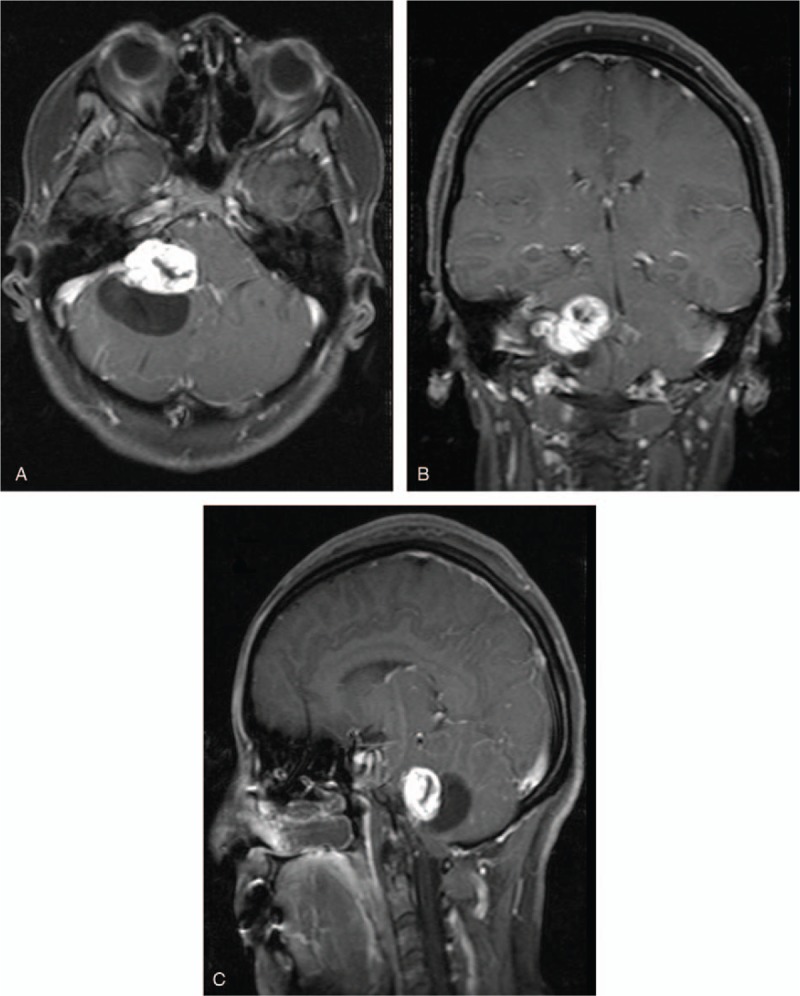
(A–C) MRIs showing a cystic-solid tumor. MRI = magnetic resonance imaging.
